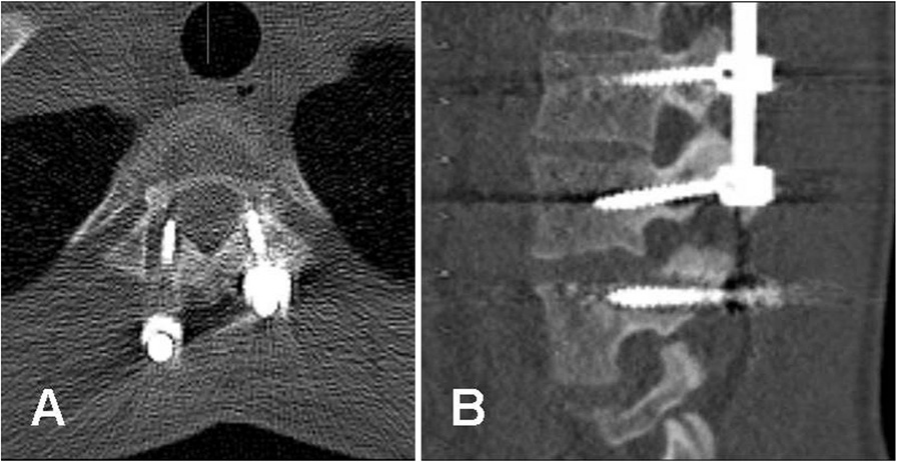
Evidence of screw loosening. CT of a 17 years old girl following segmental pedicle screw fixation T3-L3: (A-B). (A) axial image showing evidence of loosening around the screw through the right pedicle of T3 and to lesser extent around the screw through the left pedicle. (B) sagittal image at the lumbar level showing evidence of loosening around screw through the left pedicle of L3.
